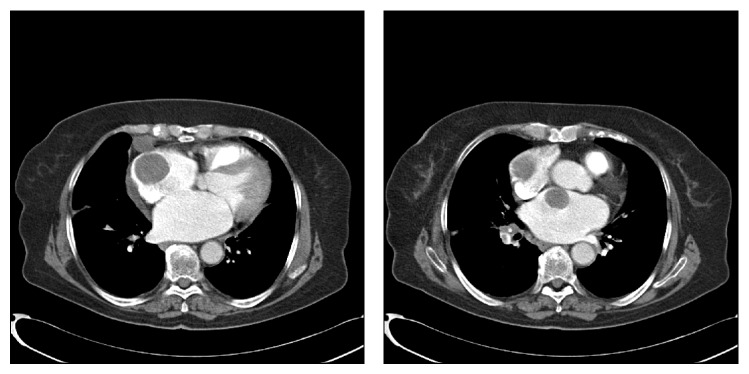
Thoracic CT demonstrates two masses with biatrial cardiac metastases of malignant melanomas in uterine cervix: one is 36 × 37 mm and the other is 26 × 20 mm in right and left atriums.
